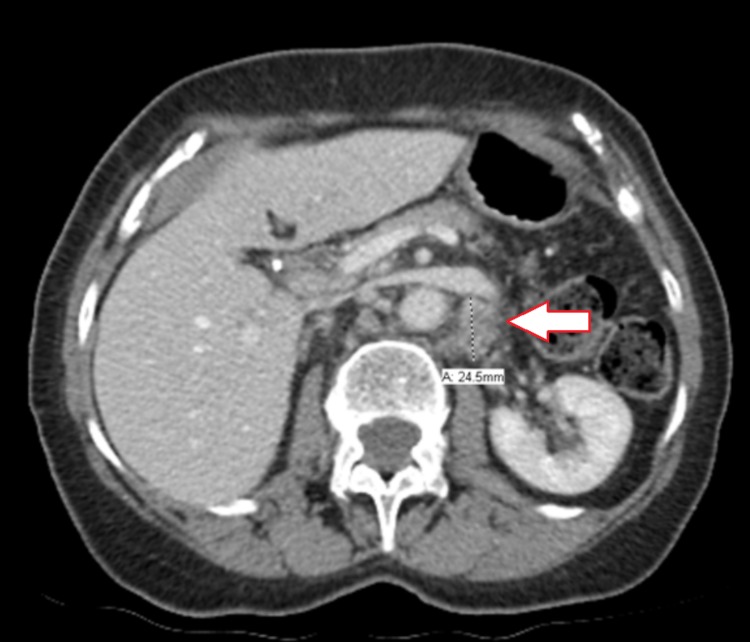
Initial computed tomography (CT) abdomen demonstrating an enlarged left peri-aortic lymph node
